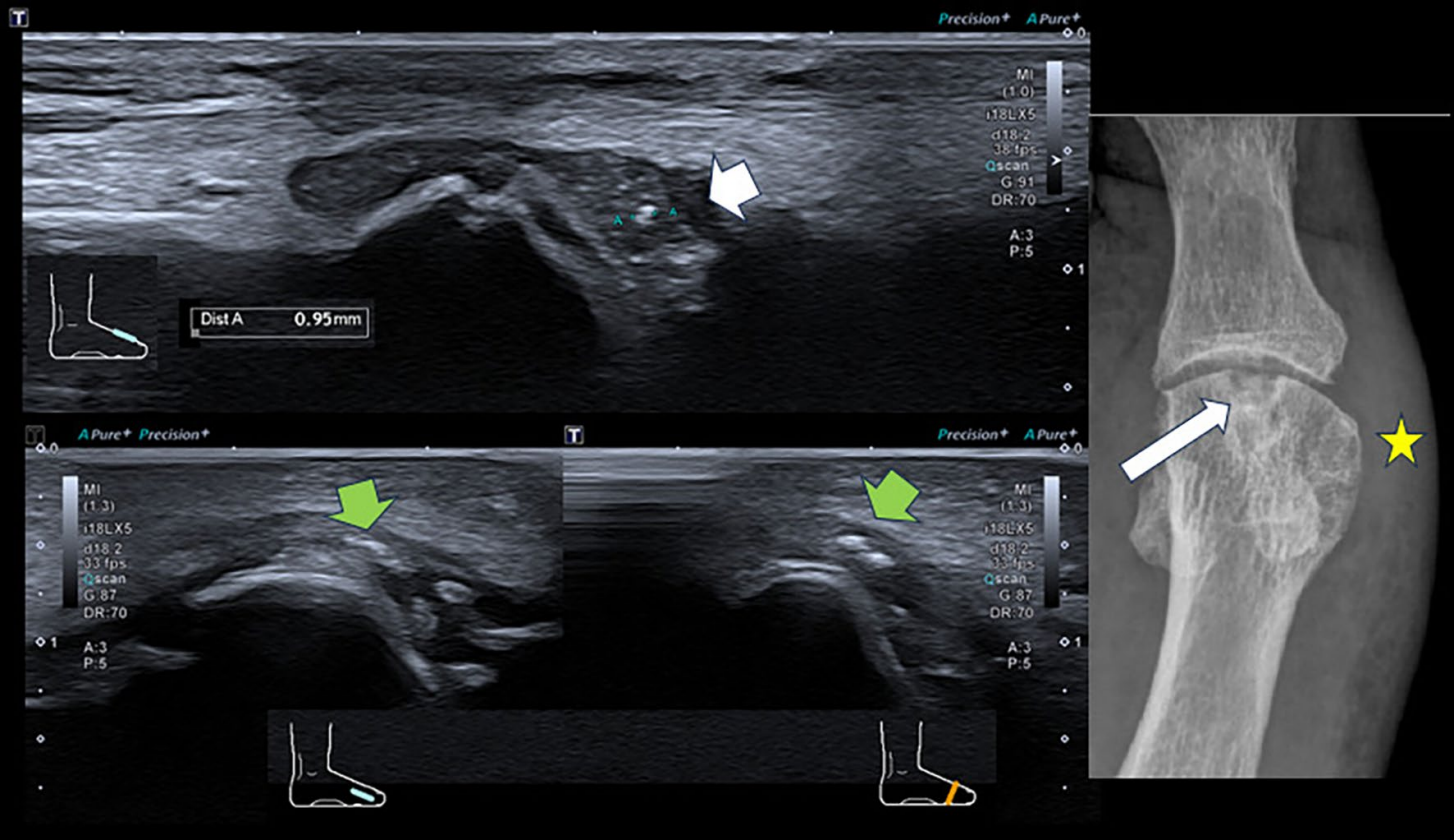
B-mode ultrasound images using an 18 MHz linear transducer and AP x-ray magnification of the first metatarsophalangeal joint of the first toe of the left foot in a 45-year-old man with a history of chronic gout presenting with an acute crisis. The long soft arrow shows a non-marginal erosion of the metatarsal head, which on ultrasound shows joint effusion, synovial thickening and internal mobile particulate content forming the ‘starry sky’ sign compatible with urate crystal deposits. The yellow star shows oedema of the superficial periarticular soft tissues
